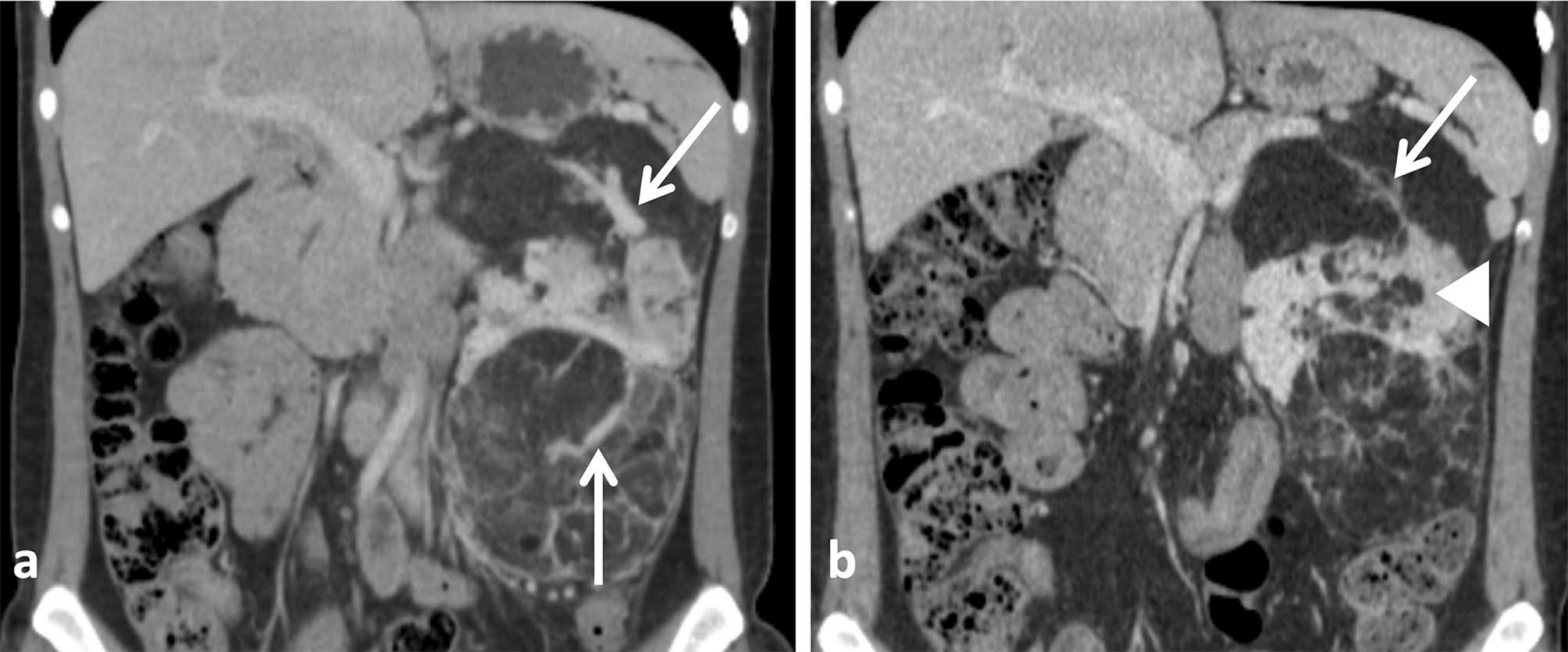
Response of intralesional vascular structures to sirolimus. Coronal nephrographic-phase CT images obtained (a) before the treatment and (b) after the treatment show disappearance of a left kidney large intralesional vascular structure and remarkable reduction of an intralesional aneurysm in response to treatment with sirolimus (arrows). An increase in the fat component is also seen (arrowhead).
